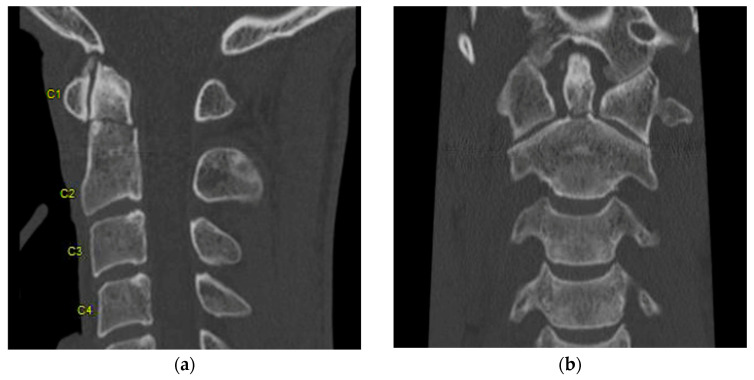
(a) Sagittal and (b) coronal view of CT showing type II dens fracture. “C1–C4” Designate each corresponding spinal level.
